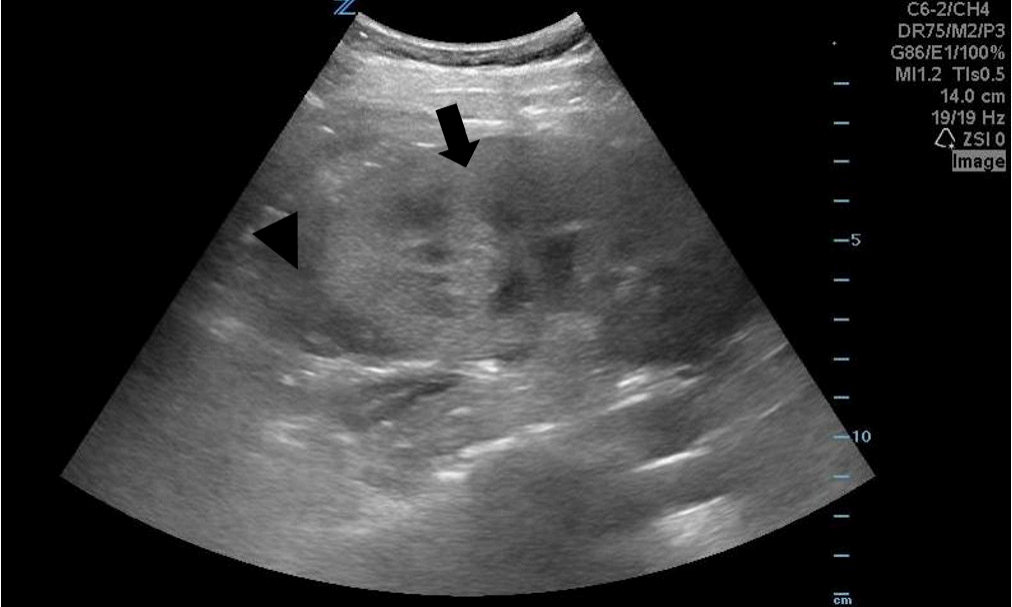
Point-of-care ultrasound of the right kidney seen in the longitudinal plane
